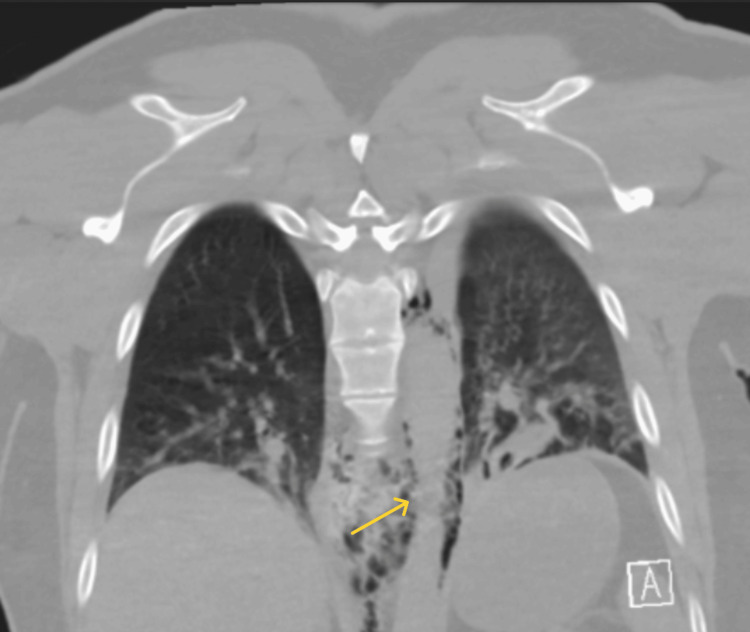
CT chest coronal view showing esophageal narrowing and perforation
